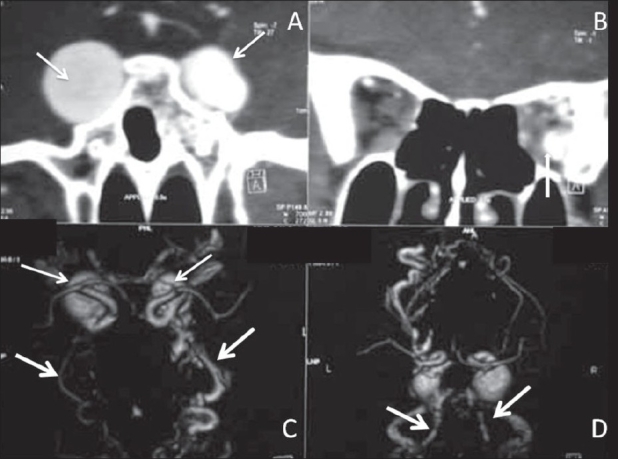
Computed tomography angiography (CTA) images. The coronal images (A, B) show bilateral cavernous internal carotid artery (ICA) giant aneurysms (arrows in A), with dilated ophthalmic veins (arrow in B). The volume rendering technique VRT images (C, D) show bilateral cavernous ICA aneurysms (arrows in C) as well as the early drainage through both the anterior ophthalmic veins (thick arrows in C) and the posterior petrous (thick arrows in D) system of veins
